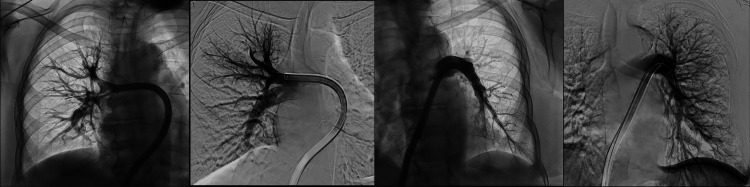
Pulmonary thrombectomy: CT images demonstrating pre-thrombectomy and post-thrombectomy results in the right and left lungs CT, computed tomography.
